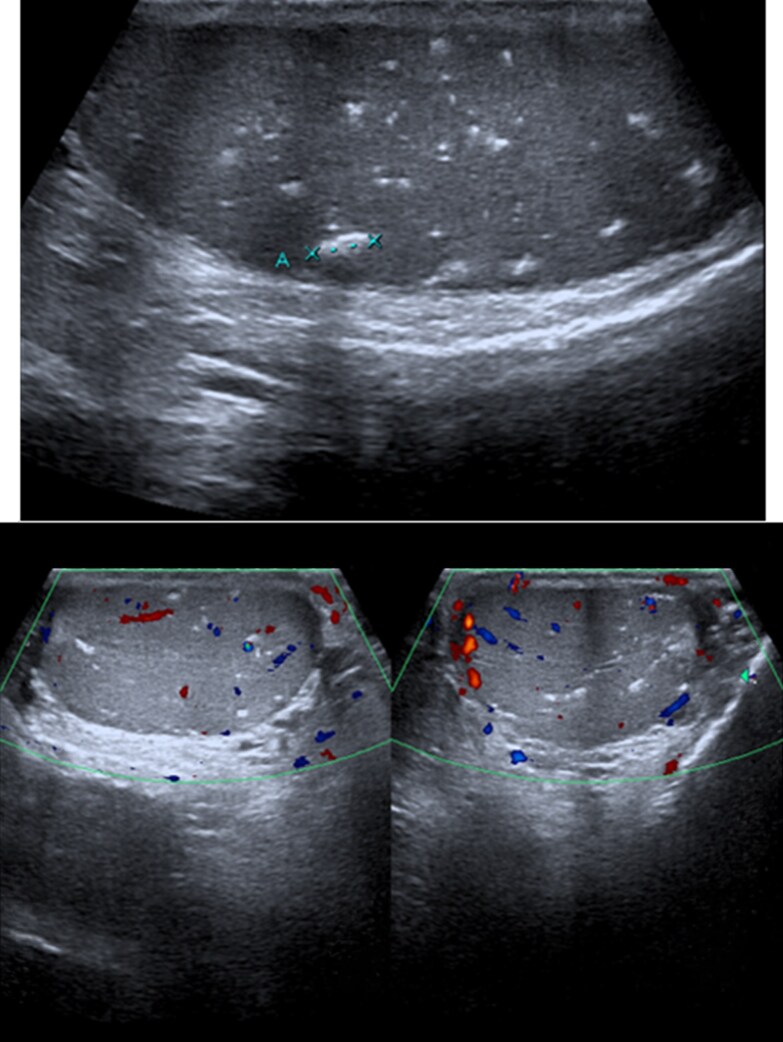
Testicular ultrasound showing microlithiasis.
